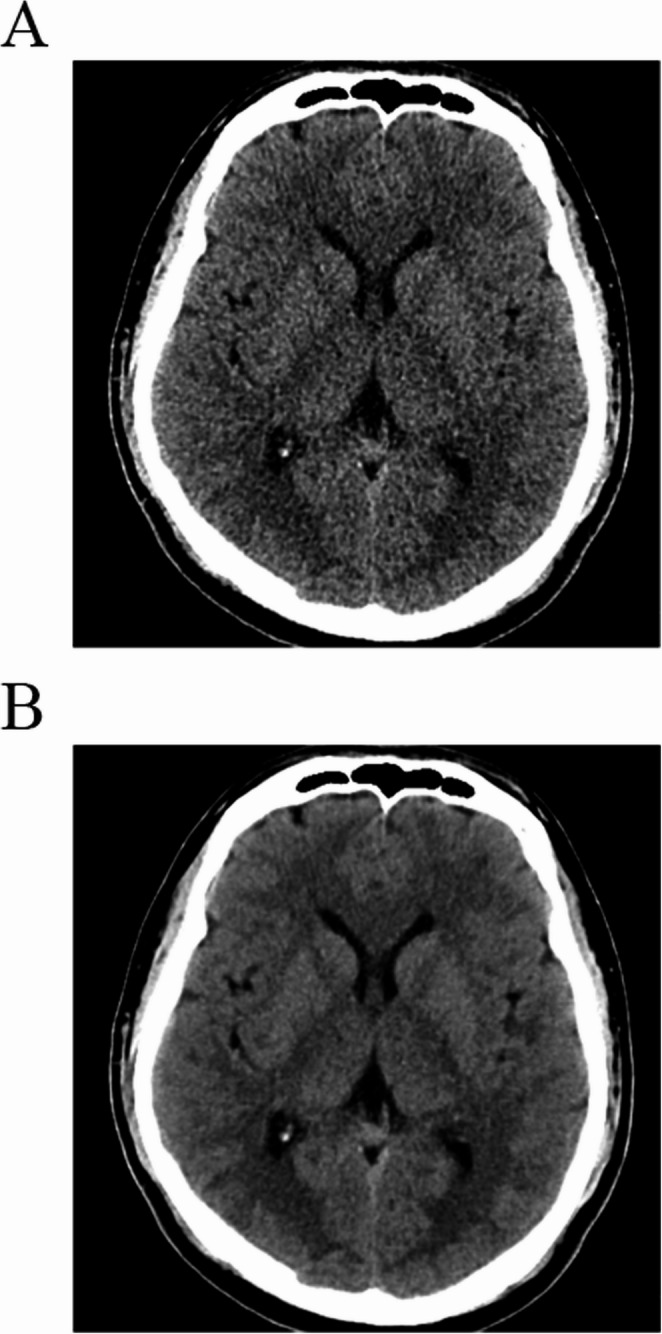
Example of two differently reconstructed normal head images from the same CT scan. Compared to vendor-provided hybrid iterative reconstruction image (adaptive iterative dose reduction, AIDR, Canon Medical Systems) (a), deep-learning reconstruction image (ClariCT.AI, ClariPi) exhibited reduced noise and improved delineation of gray-white matter boundaries (b)
